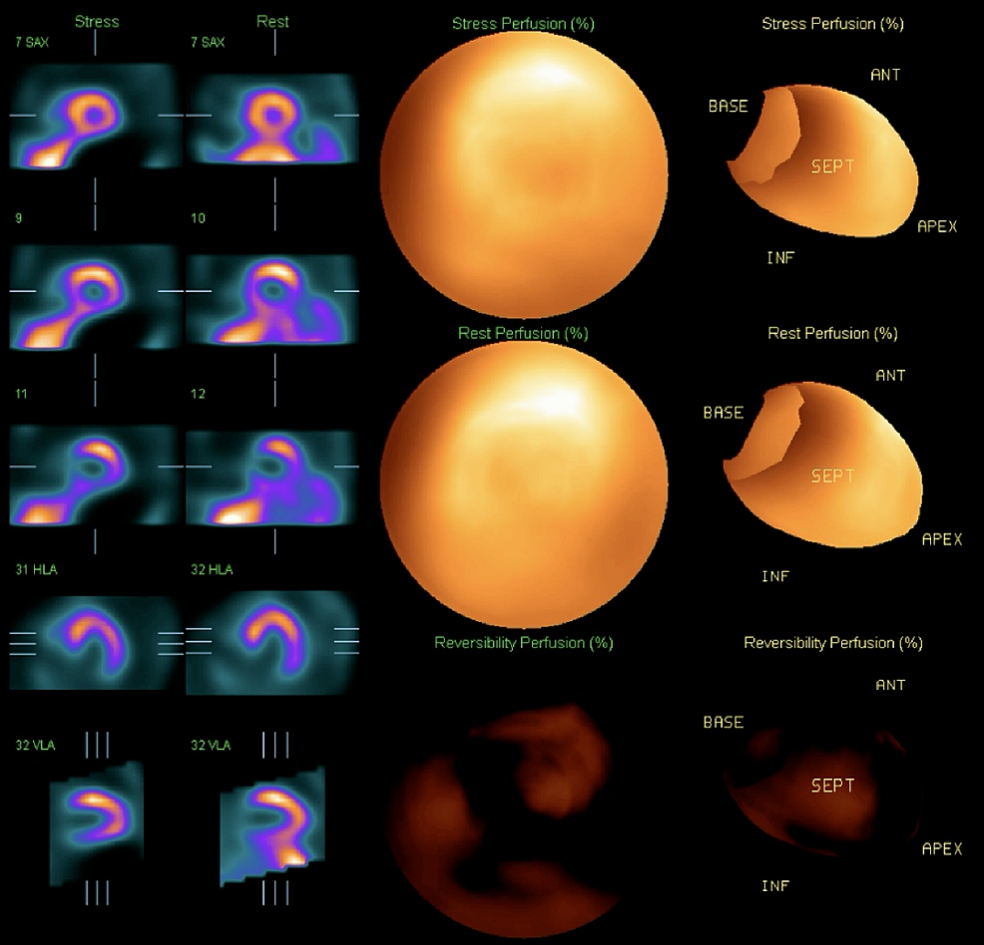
SPECT image 2 showing a small to moderate reversible perfusion defect present in the mid and basal aspect of the inferoseptal wall of the left ventricle. SPECT: single photon emission computed tomography.
